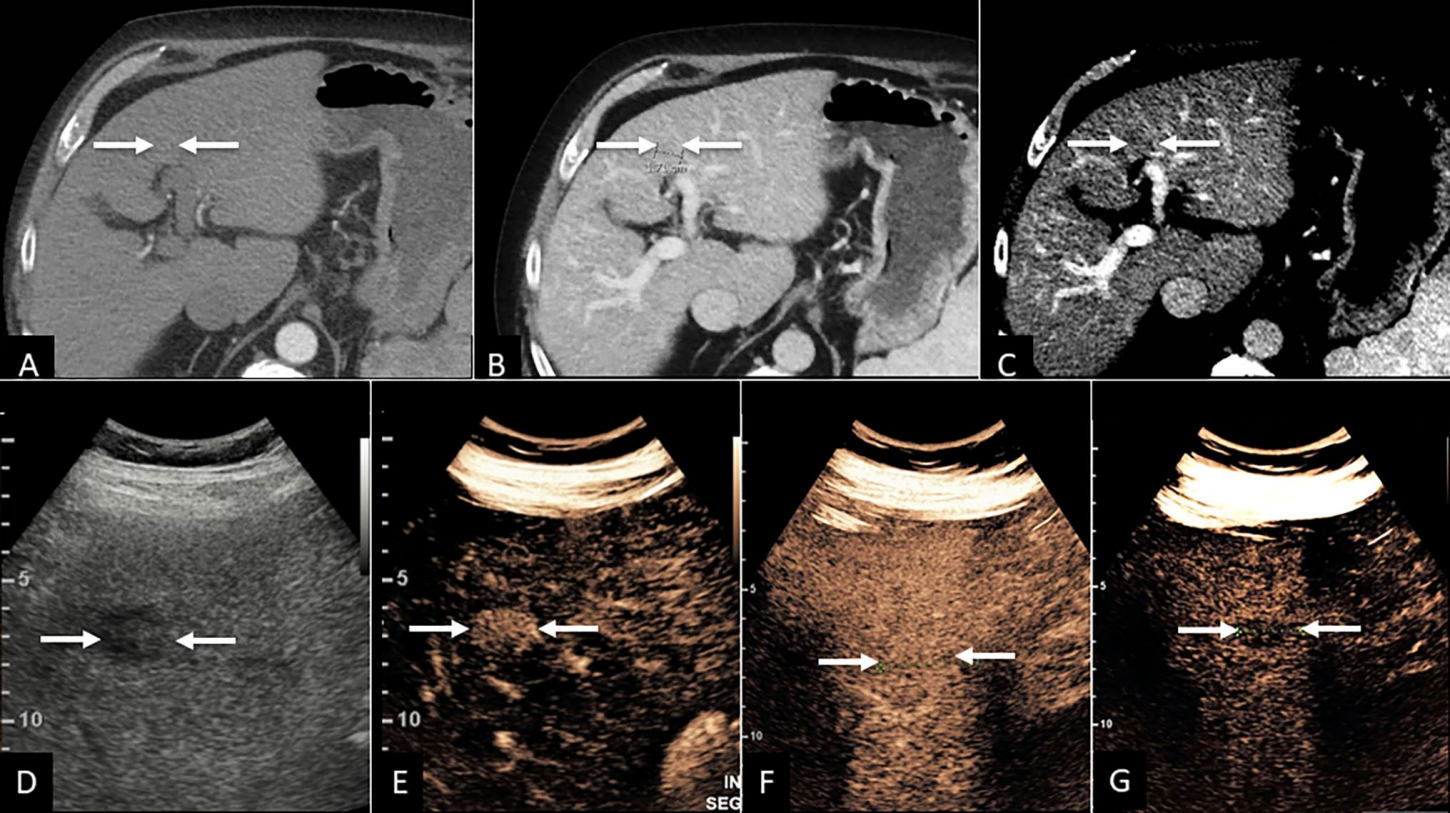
HCC with CT LR-3 categorization. A Arterial phase CT image demonstrating small nodule with no APHE (arrows). Later phase images (B, C) demonstrated barely perceptible washout. D Grayscale ultrasound image demonstrating hypoechoic nodule in a fatty liver measuring 2.5 cm with clear APHE (E). CEUS representative image at 1 min (F) showing no early washout. G CEUS representative image at 3 min demonstrating late and mild washout—typical appearance of HCC (LR-5), confirmed on biopsy
